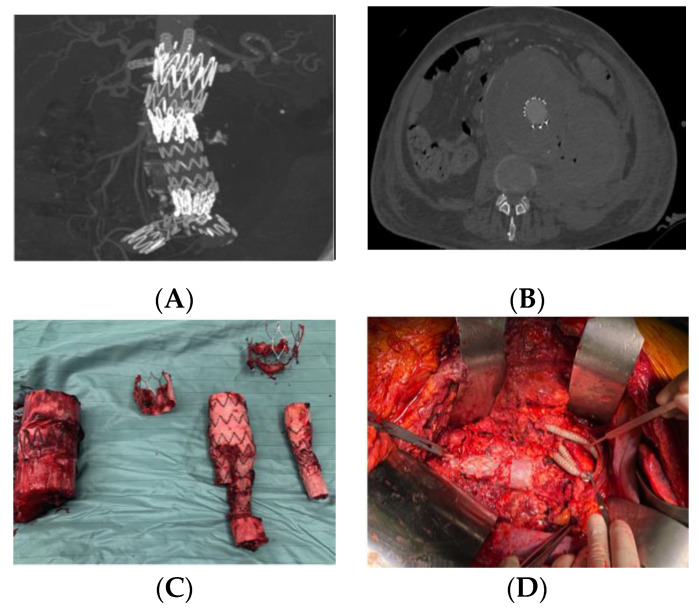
(A,B) Pre-operative computed tomography (CT) scan showing graft disruption, perigraft fluid and air in a 72-year-old man with an infected abdominal endograft; (C) explanted graft after in situ repair (ISR) with visceral debranching: aorto-mesenteric bypass, right renal artery Y graft, aorto-left renal artery bypass. The reconstruction has been completed with lower extremity revascularization with extra-anatomic reconstruction (EAR) axillo-bifemoral; (D) final result after EAR.
